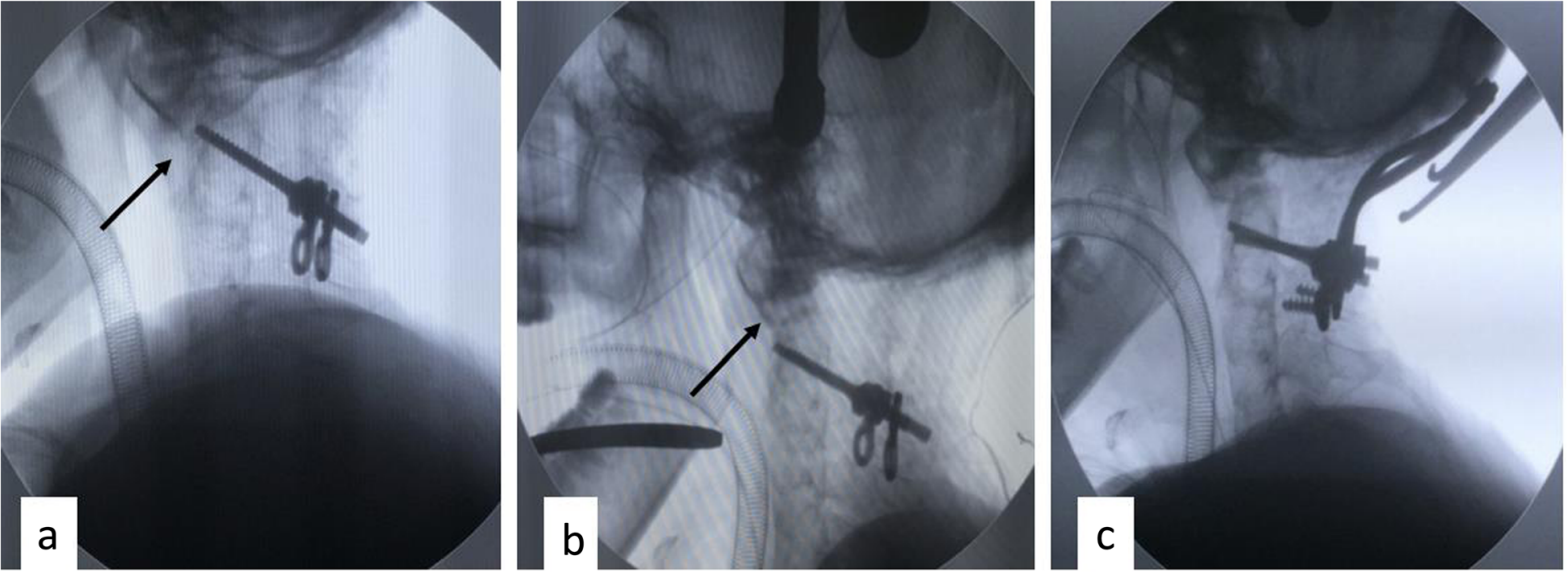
Intraoperative C-arm radiograph in the revision surgery. a C2 pedicle screws were inserted. b After anterior transoral release, the tip of the odontoid was clearly brought down. c The posterior occipitocervix fixation was performed
